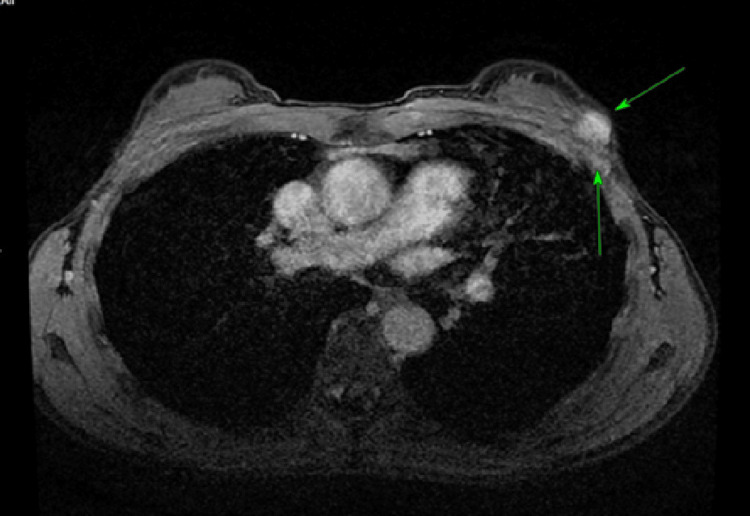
(Case 6) Axial plane MRI breasts shows left breast carcinoma.
